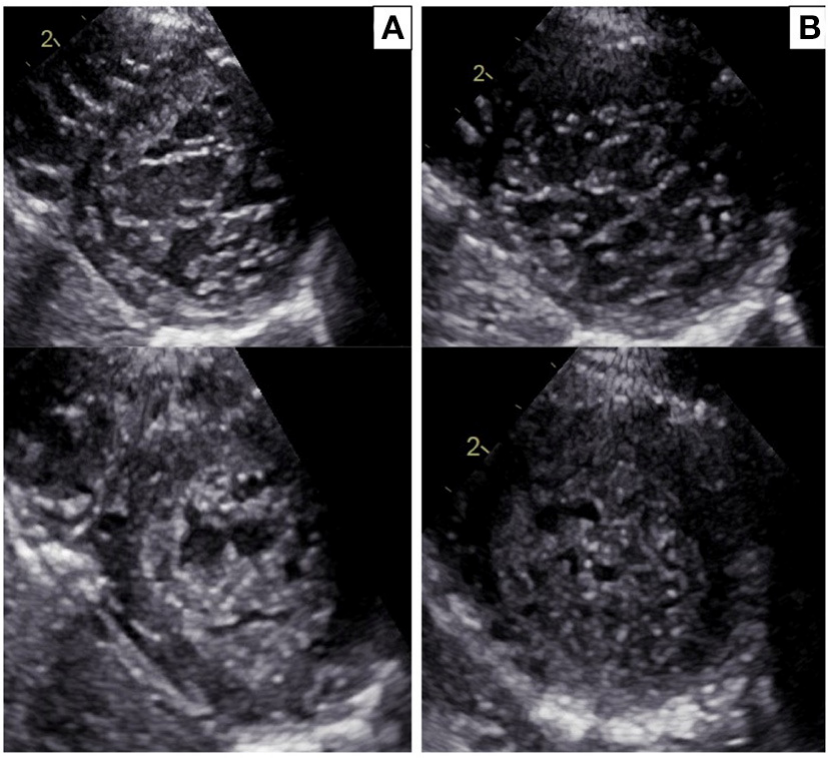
(A) Echocardiographic parasternal short-axis view in the proband: diastole (upper panel) and systole (bottom panel). Trabecular/compacted end-systolic wall thickness ratio >2 (positive Jenni criteria for LVNC). (B) Echocardiographic parasternal short-axis view in the sister of the proband: diastole (upper panel) and systole (bottom panel). Trabecular/compacted end-systolic wall thickness ratio >2 (positive Jenni criteria for LVNC).
